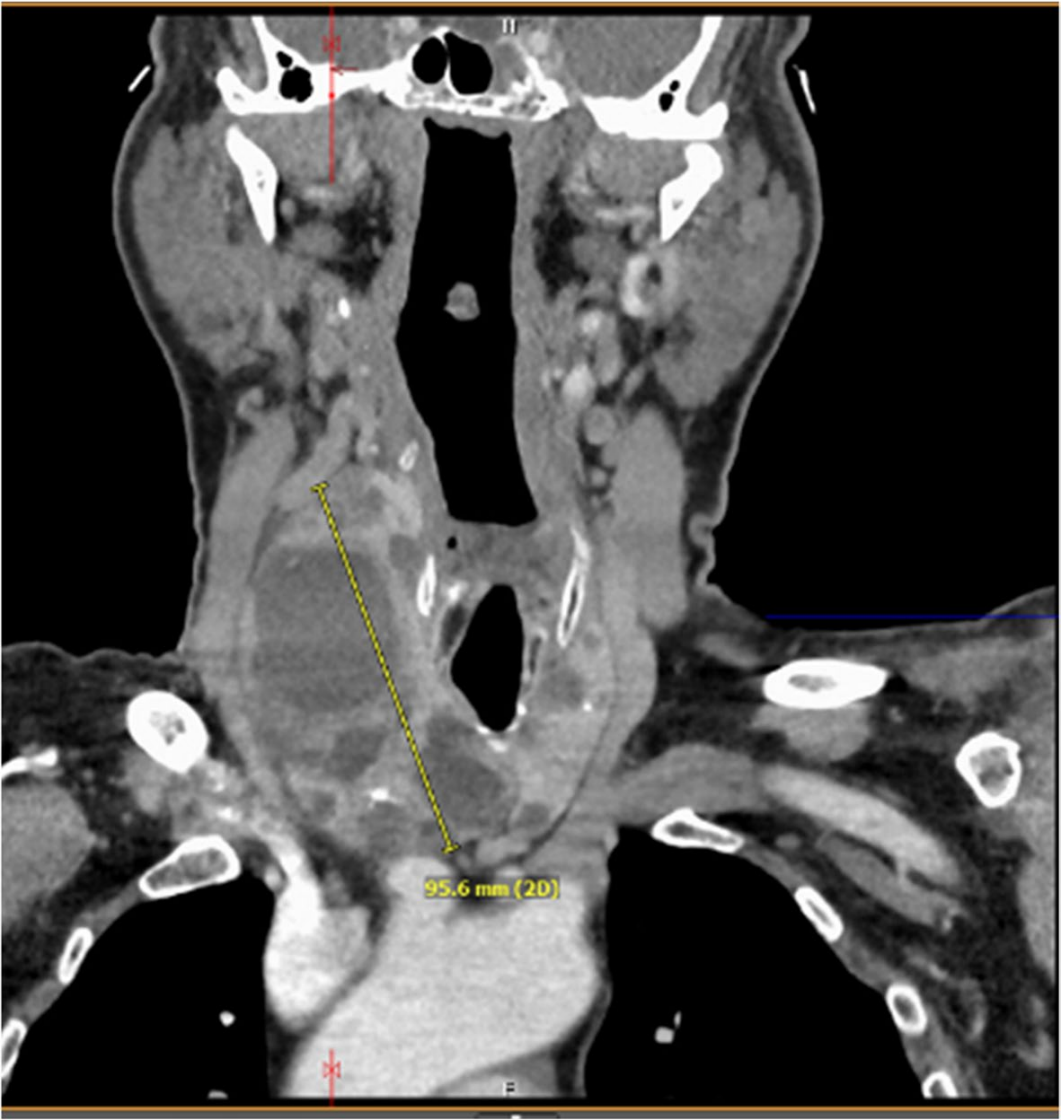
Cervical computed tomography. Enlarged right thyroid lobe (AP, W, CC) of 5.5 × 6.0 × 10.0 cm compared to the left lobe with multiple cystic and complex nodules. Extends into the upper substernal region displacing the great vessels, 60% compression of the trachea, with 1.8 cm leftward deviation of the trachea
